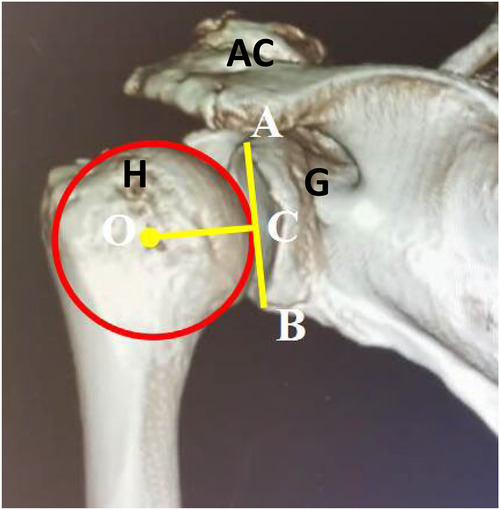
Three-dimensional CT evaluation of the displacement distance of the proximal humeral head.adjust the three-dimensional CT image of the affected shoulder to the right position of the glenohumeral joint. View from the back to avoid the coracoid process blocking the field of view and fully reveal the glenoid fossa. O: center of the humeral head; A: superior edge of the glenoid fossa; B: inferior edge of the glenoid fossa; C: vertical foot passing through the center of the humeral head and perpendicular to the glenoid fossa line; the distance between C and B represents the height of the center of the humeral head relative to the inferior edge of the glenoid fossa (H, humeral head; AC, acromioclavicular joint; G, Glenoid).
